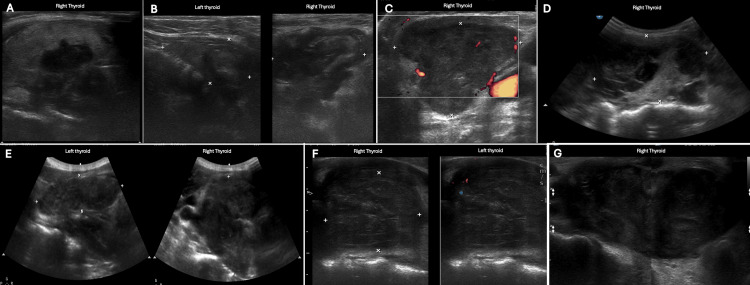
Ultrasound findings (A) The right is almost totally replaced by a large heterogeneous, hypoechoic lesion with evident vascularity and irregular outline. (B) Diffuse enlargement of the thyroid gland with ill-defined margins and possible retrosternal extension, showing heterogeneous echotexture with multiple variable-sized nodules. (C) Heterogeneous mass lesion, mainly hypoechoic, occupying most of the right thyroid lobe and showing minimal internal color flow. (D) Enlarged right lobe of the thyroid gland noted with a complex cystic nodule having internal echoes. (E) Both thyroid lobes are diffusely markedly enlarged and heterogeneous with multiple hypoechoic small nodules. (F) Large heterogeneous nodule seen occupying and enlarging the left thyroid gland. (G) Enlarged right thyroid lobe and isthmus, nearly totally occupied by a large heterogeneous hypoechoic nodule.
